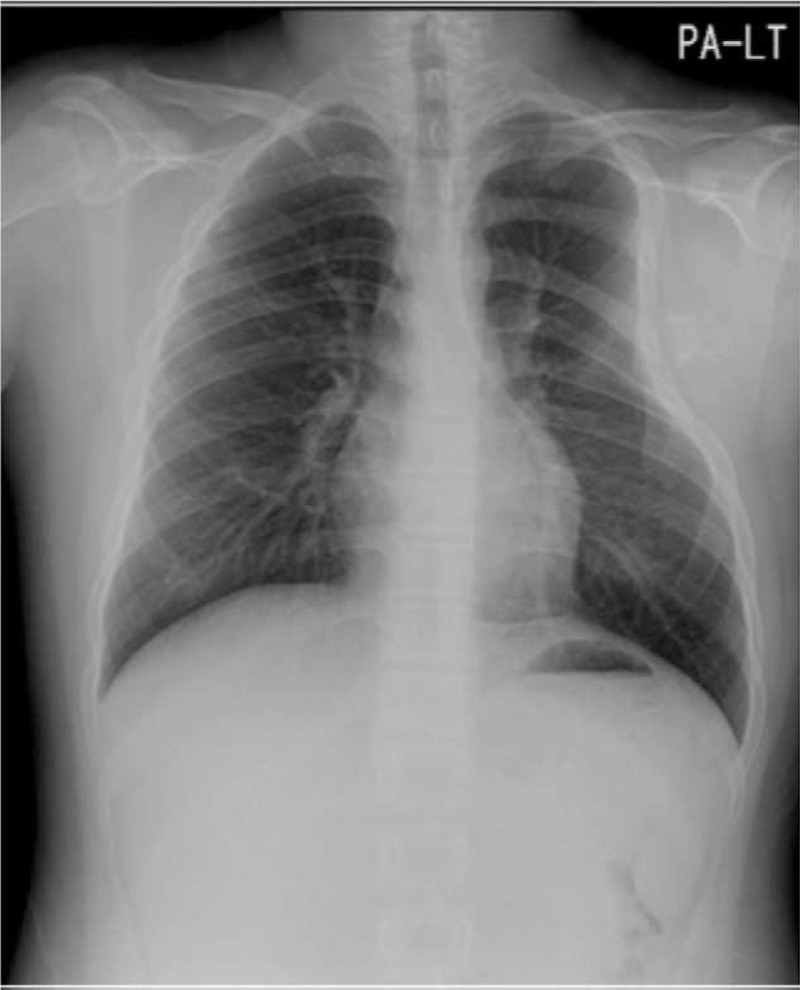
A 1-year follow-up chest anteroposterior radiographs shows no recurrence of osteochondroma and improvement of compression of chest wall.
